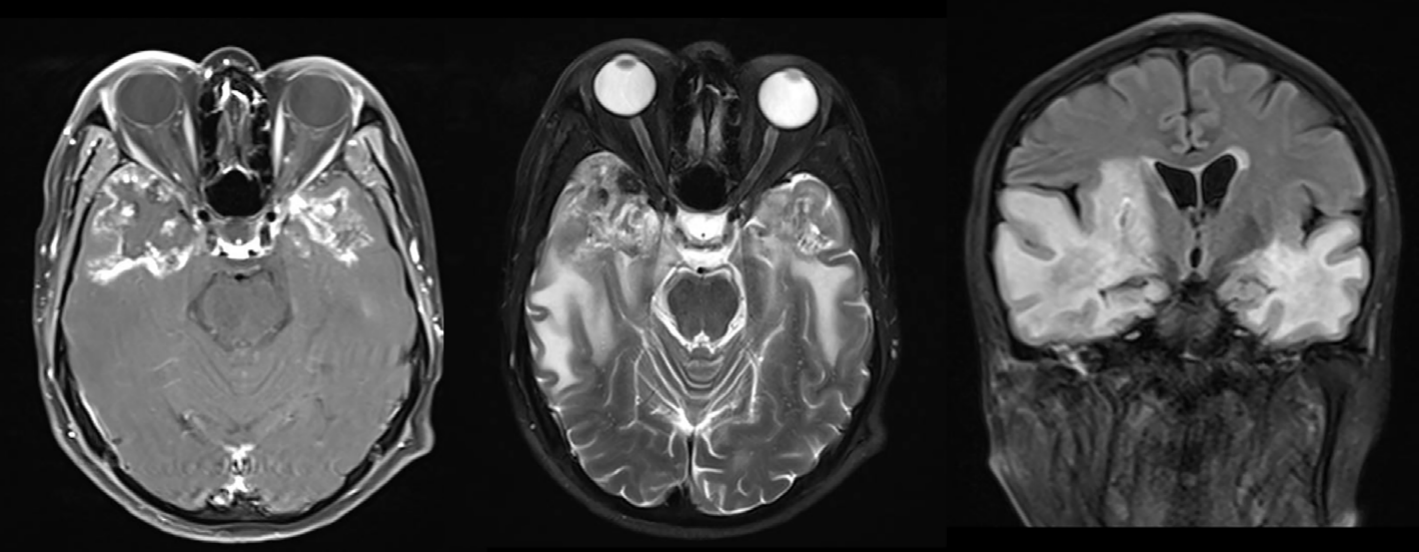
Typical MR images of radiation-induced temporal lobe injury (TLI).
